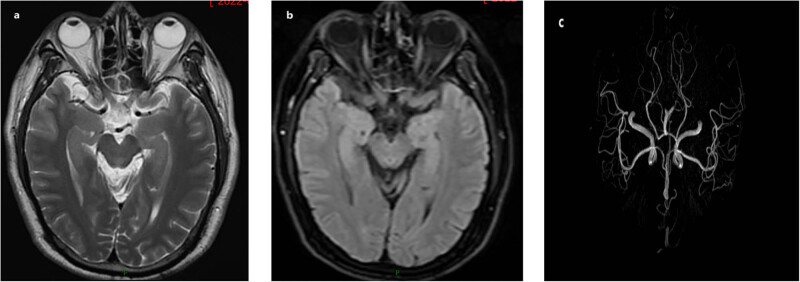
Head MRI and MRA of the patient. (a) and (b) Cranial MRI showed bilateral frontal lobe ischemia near bilateral lateral ventricles. (c) No obvious vascular stenosis was found on MRA.
